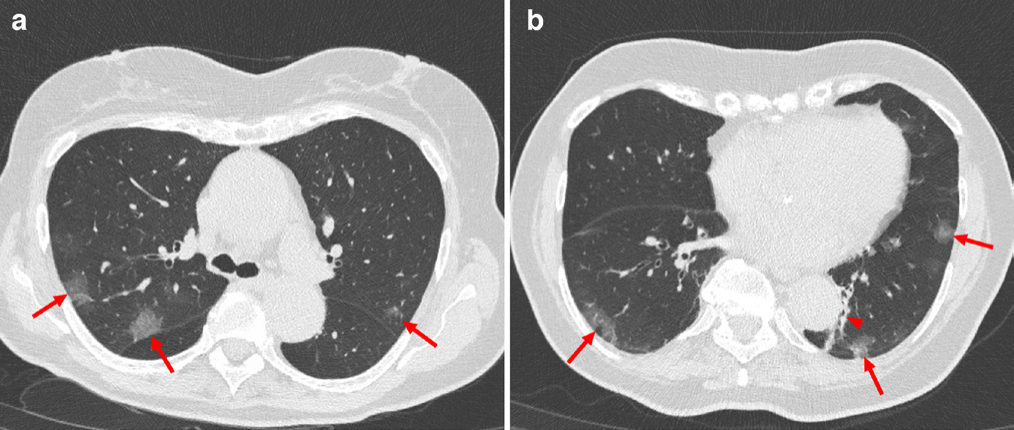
Typical CT features of peripherally distributed multifocal ground-glass opacities (red arrows) with posterior part (A, B)/lower lobe (B) pre-dilection in a 81-year-old female patient with COVID-19 who presented with presented with dyspnea and fever. Note; presence of atelectatic changes lateral from the descending aorta (arrowhead in B) and presence of minor respiration artifacts in the left lower lobe.
